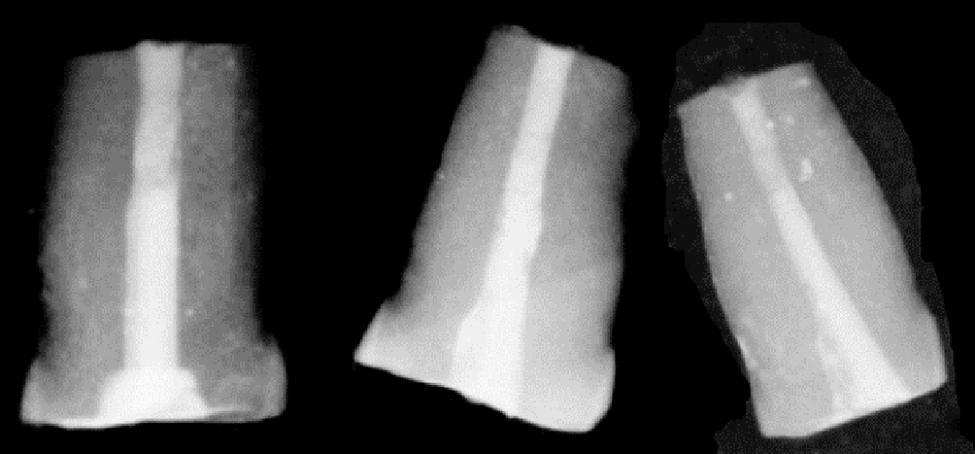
Radiographic view of the roots following the application of MTA and CC mixed with blood in the root-end cavity
